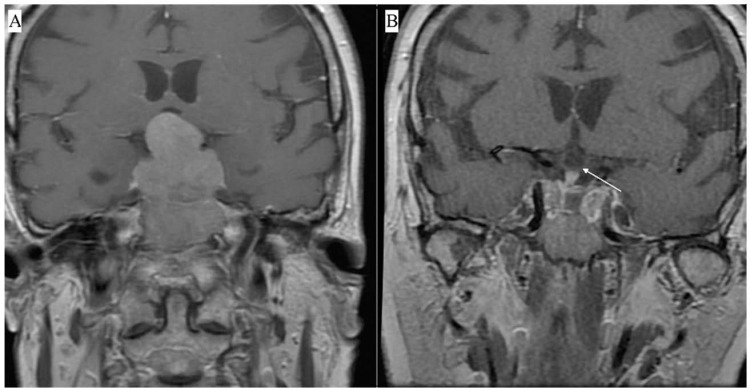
Contrast-enhanced MRI showing a macroadenoma penetrating into the suprasellar region and compressing the optic chiasm. Preoperative T1—weighted contrast-enhanced coronal view (A), postoperative T1—weighted contrast-enhanced coronal view. The white arrow indicates the optic chiasm visible after tumor resection (B).
